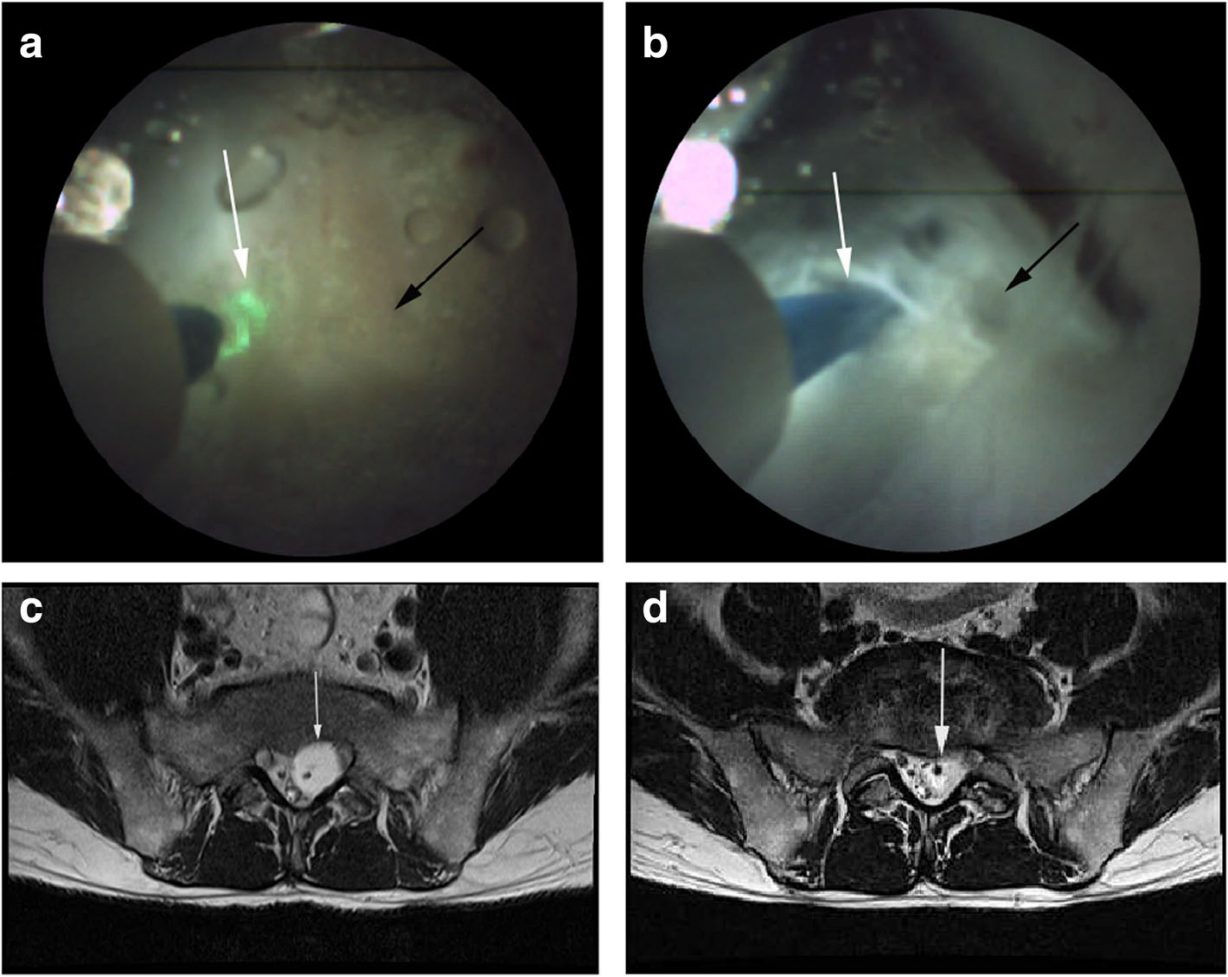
a Epiduroscopic image shows a laser electrode inserted into the cystic mass (white arrow) and the confirmed mass (black arrow). b Epiduroscopic image shows a laser electrode inserted into the cystic mass (white arrow); after decompression, cerebrospinal fluid was expelled and the cyst decompressed (black arrow). c T2-weighted axial magnetic resonance (MR) image shows the inflated cyst containing cerebrospinal fluid and occupying the spinal canal (white arrow). d Post-operative MR image shows the released dural sac and deflated Tarlov cyst (white arrow)
